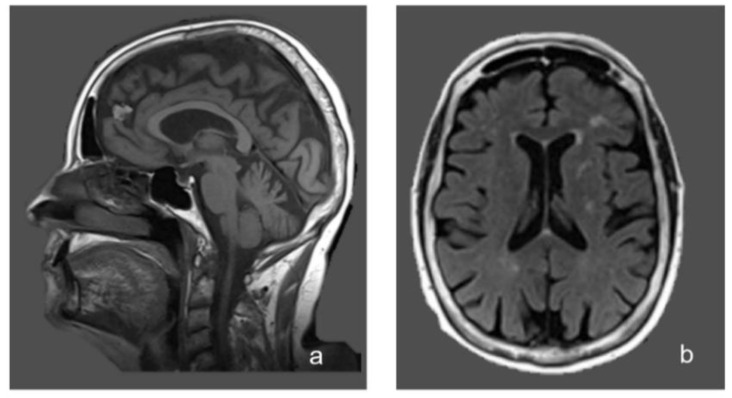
Sagittal (a) and axial (b) magnetic resonance imaging (MRI) Fluid-Attenuated Inversion Recovery (FLAIR) images of the patient, showing multiple areas of white matter hyperintensity and cerebellar atrophy.
